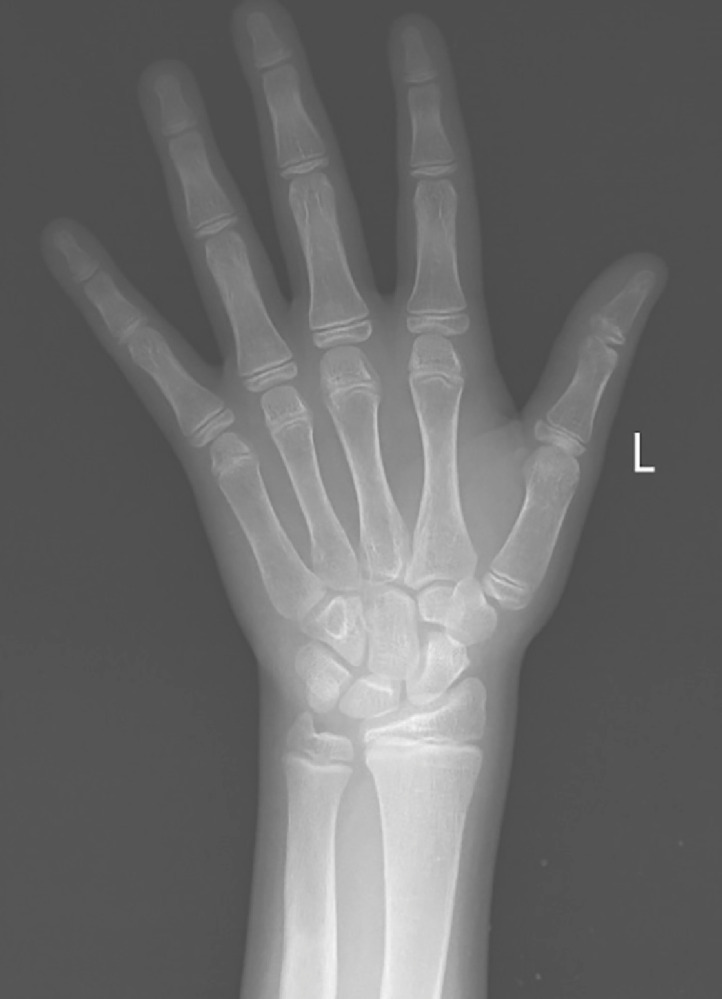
X-ray of the left hand shows the bone age matches the reference standard of 13 years with a standard deviation of +/-12 months (according to Greulich and Pyle atlas). At that time, the patient's chronological age was 6 years (indicating significant advanced bone age).
